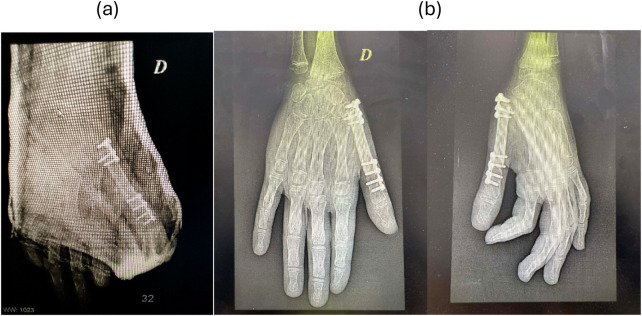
Postoperative radiograph (a) and at one-year follow-up (b).
